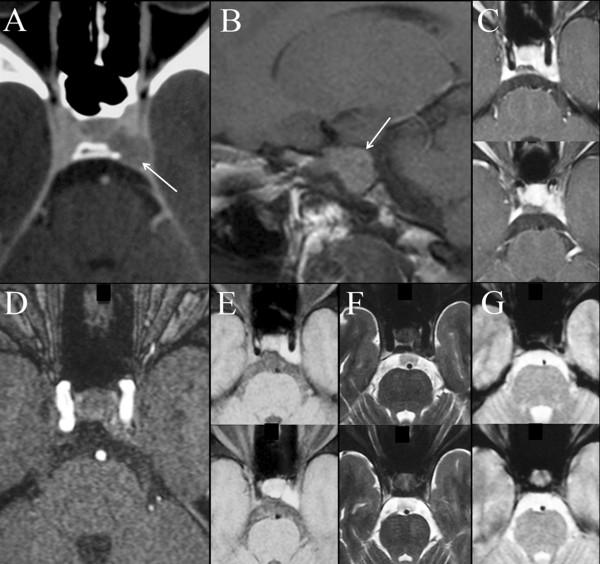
Neuroradiological findings at diagnosis of the lesion inside the cavernous sinus. Contrast enhanced CT (A), documenting the space occupying lesion in the left cavernous sinus. T1-weighted images documenting the lesion, which is spontaneously hyper-intense (sagittal view; B – white arrow) and has scarce contrast-enhancement (fat suppressed turbo spin echo T1-weighted axial images after contrast enhancement; C). Axial source image from MR-angio ruled out a vascular lesion (D). The mass lesion remained hyper-intense on un-enhanced fat-saturation T1-weighted images (E), strongly hypo-intense on turbo-spin echo fat-saturated T2-weighted images (F), and iso-intense on gradient echo T2*-weighted images (G).
